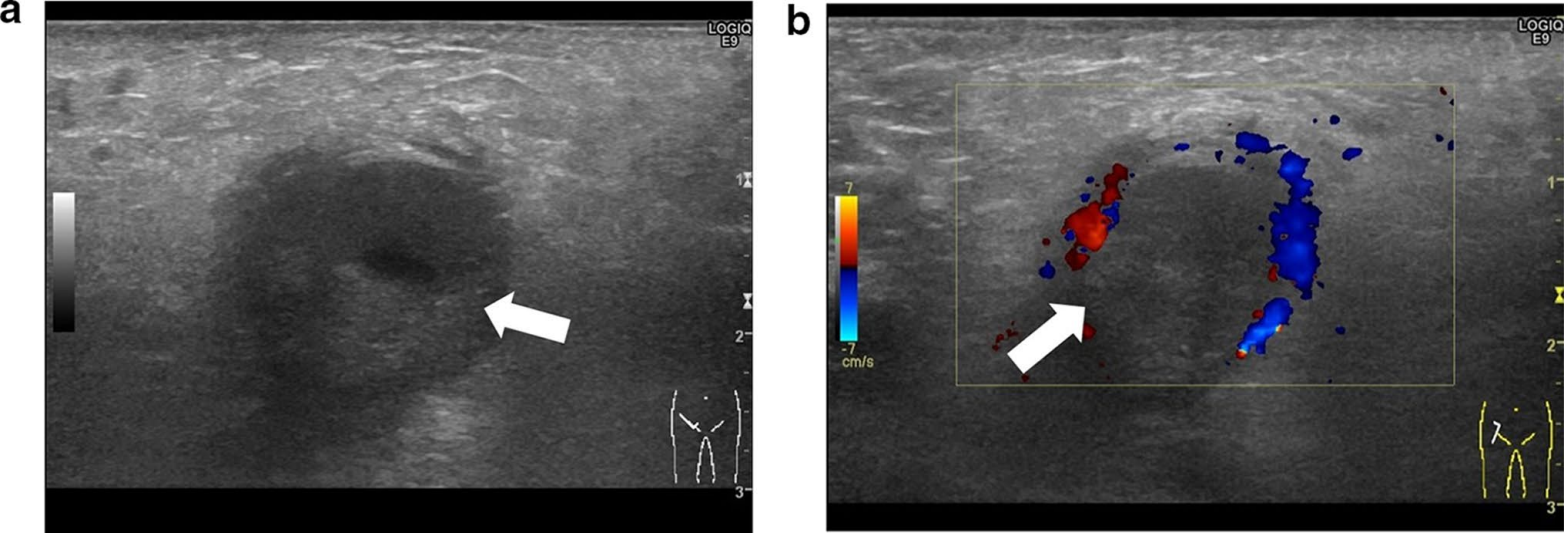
Ultrasonographic images of a 11-month-old baby with undescended testicular torsion. a Ultrasonography shows swelling of the inguinal soft tissue, and low-echoic testis is evident. b Color Doppler sonogram shows absence of blood flow within the undescended testis
